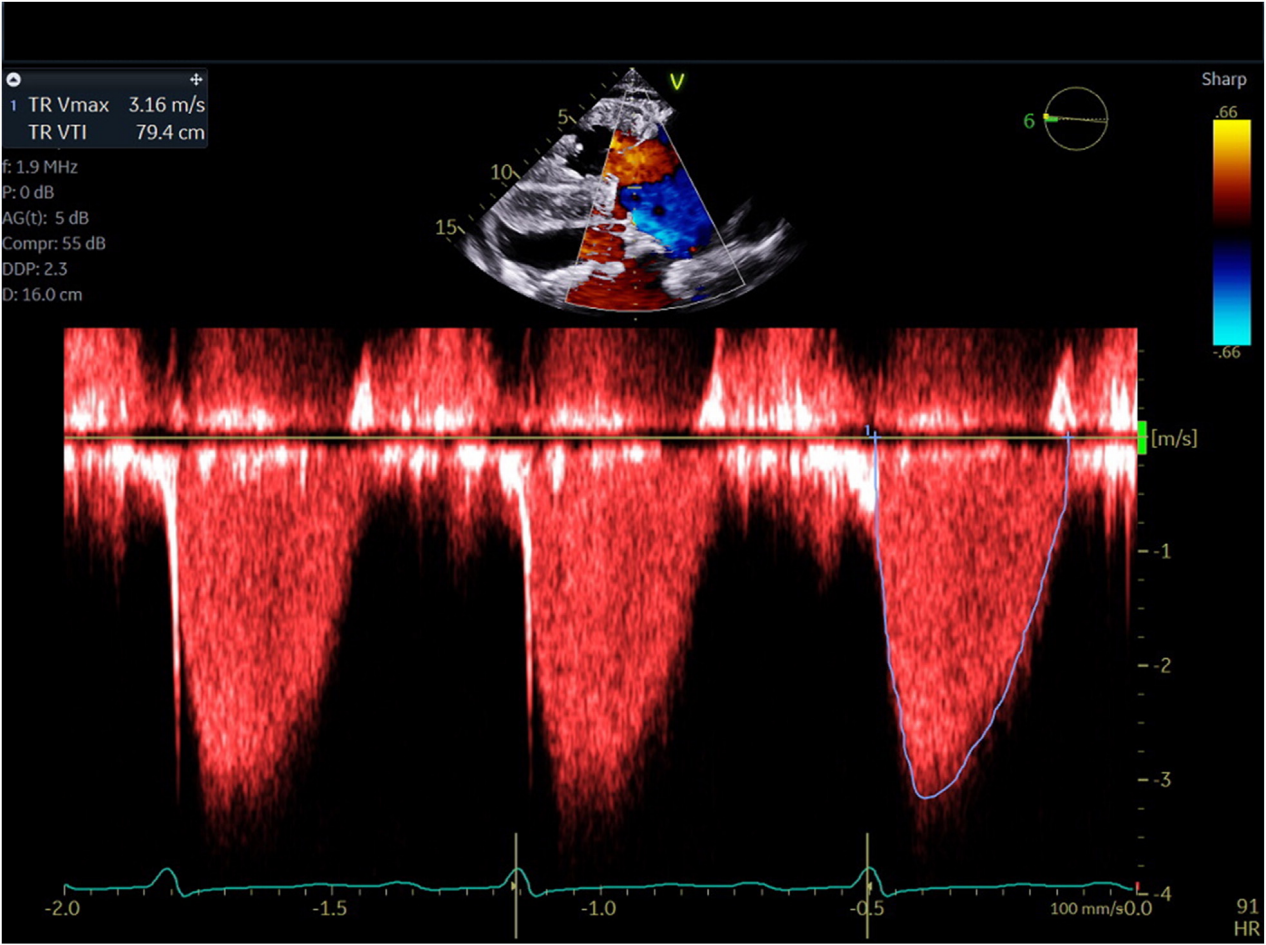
Patient 2 preintervention transthoracic echocardiogram demonstrating torrential tricuspid regurgitation and flail leaflet.
